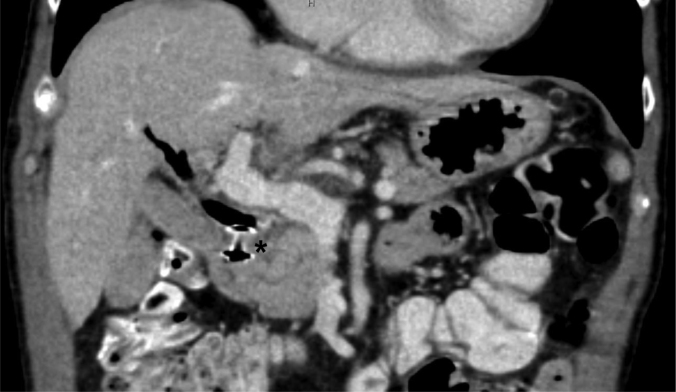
Follow-up CT shows good CDS stent position (*asterisk*) in the asymptomatic patient.
